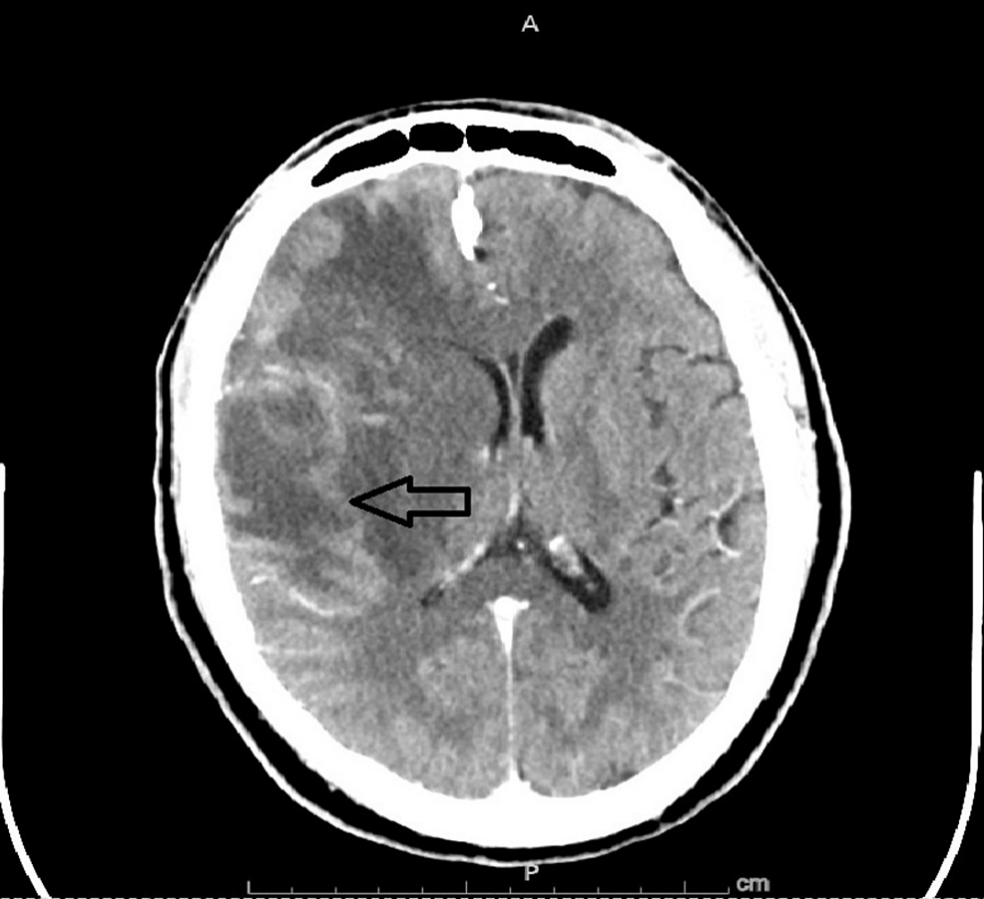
Initial CT of the head The CT of the patient's head shows a large right sided, mainly peripherally enhancing mass surrounded by large vasogenic edema (arrow).
